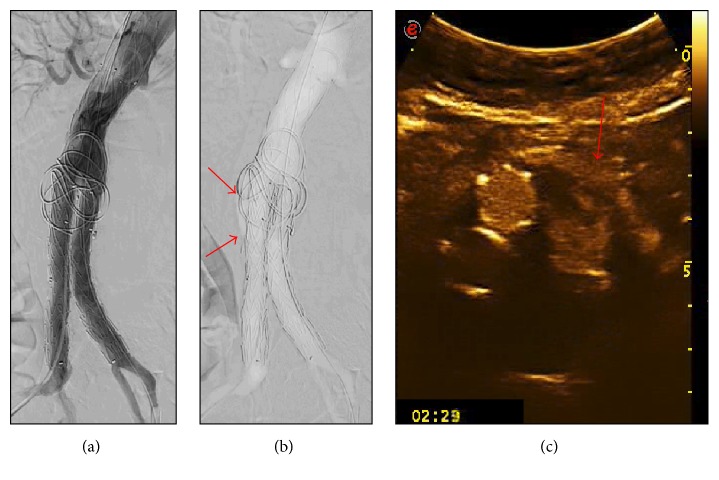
(a) Iodinate contrast media completion angiography that shows the good positioning of the suprarenal fixation endograft and the absence of endoleaks. (b) Carbon dioxide completion angiography that shows the good positioning of the suprarenal fixation endograft and the presence of ELII (as indicated by the red arrows). (c) Contrast-enhanced ultrasound that shows the presence of ELII with the inflow from inferior mesenteric artery (as indicated by the red arrow).
